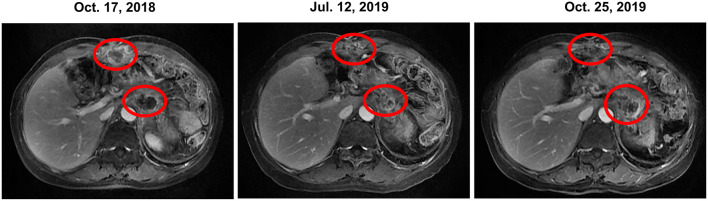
Change in the tumor prior to, during and following olaparib therapy based on representative MRI. In October 2018, prior to olaparib therapy, MRI showed anterior abdominal wall mass and retroperitoneal mass. Continuous stable disease was observed for 12 months during olaparib (with or without irinotecan) therapy. However, the retroperitoneal mass enlarged in October 2019, meanwhile, the CA19-9 level increased dramatically. Thus, the patient was transferred to SOX chemotherapy. CA19-9, carbohydrate antigen 19-9; MRI, magnetic resonance images; SOX, S-1 plus oxaliplatin.
